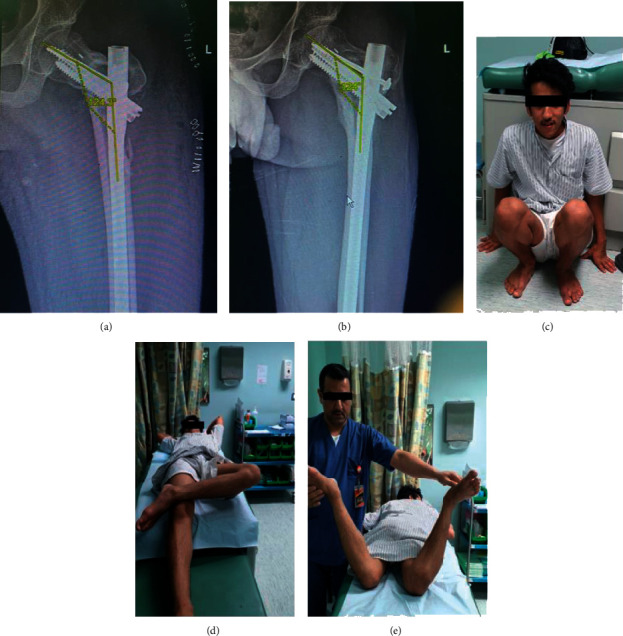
(a) Postoperative radiograph sequence showing correction achieved and the position of implant within the femoral head and intramedullary canal. (b) 6 months postoperative follow-up showing 124 degree neck-shaft angle. (c) 4 months postoperative clinical photo showing the ability of the patient to squat. (d) External rotation (cross leg). (e) Internal rotation.
